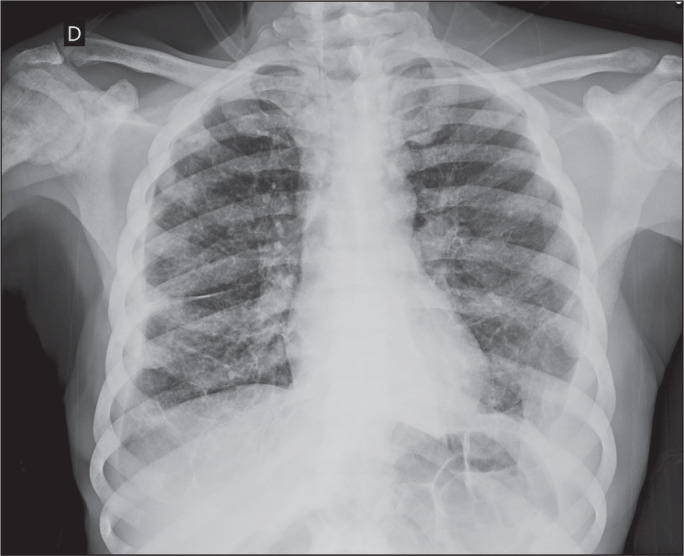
Chest X-ray obtained for investigation of anemia and wasting syndrome
